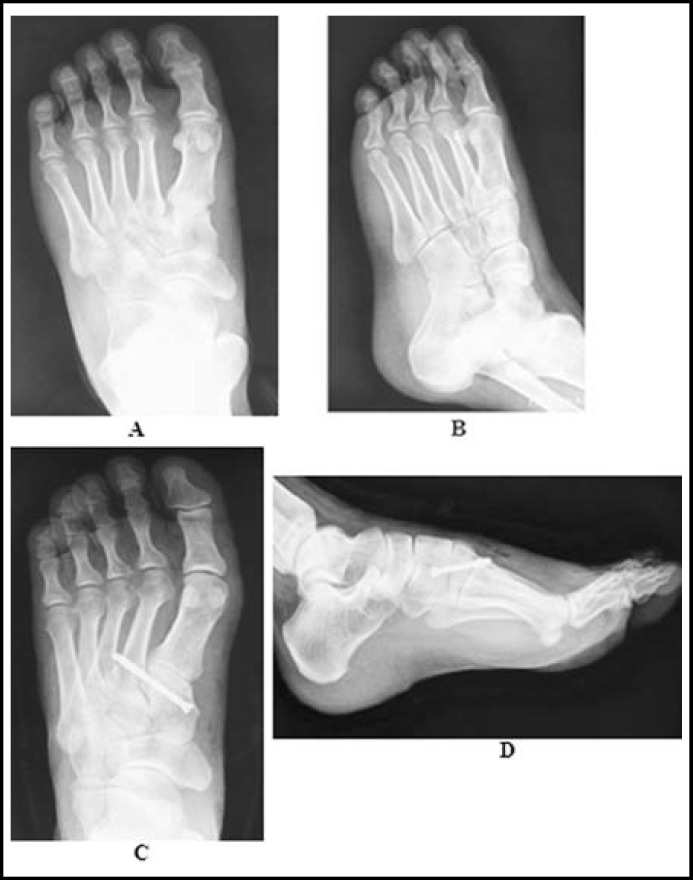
A female patient with second tarsometatarsal joint fracture dislocation in left foot caused by fall from height. (A, B) The X-ray showed a Myerson type B2 injury with the widen space between the base of first and second metatarsals. (C, D) The postoperative X-ray showed a good reduction and fixation
